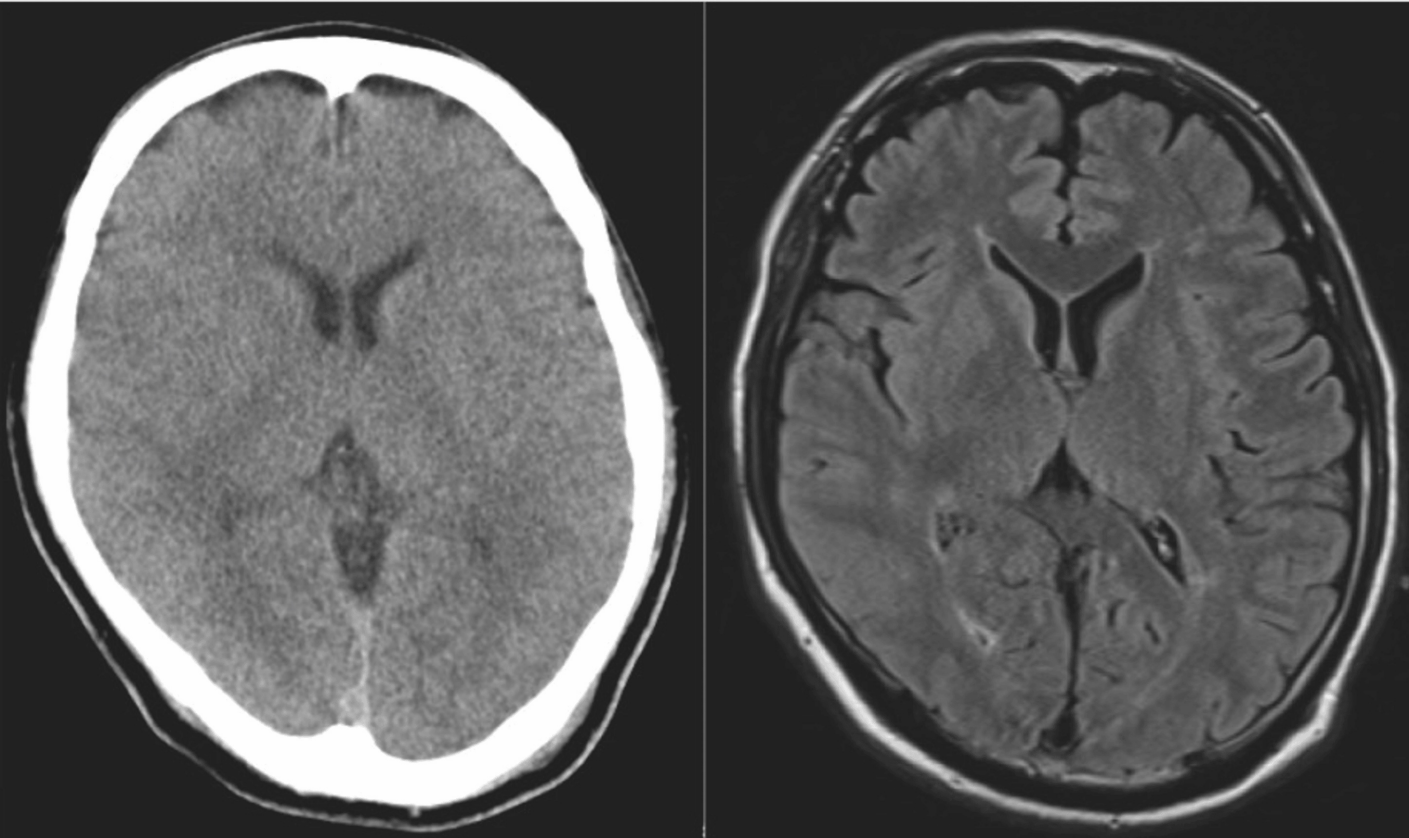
Plain head CT scan and contrast-enhanced T1-weighted MRI A plain head CT scan (left) and contrast-enhanced T1-weighted MRI (right) performed on the patient's arrival at the hospital showed no obvious cranial abnormalities.
